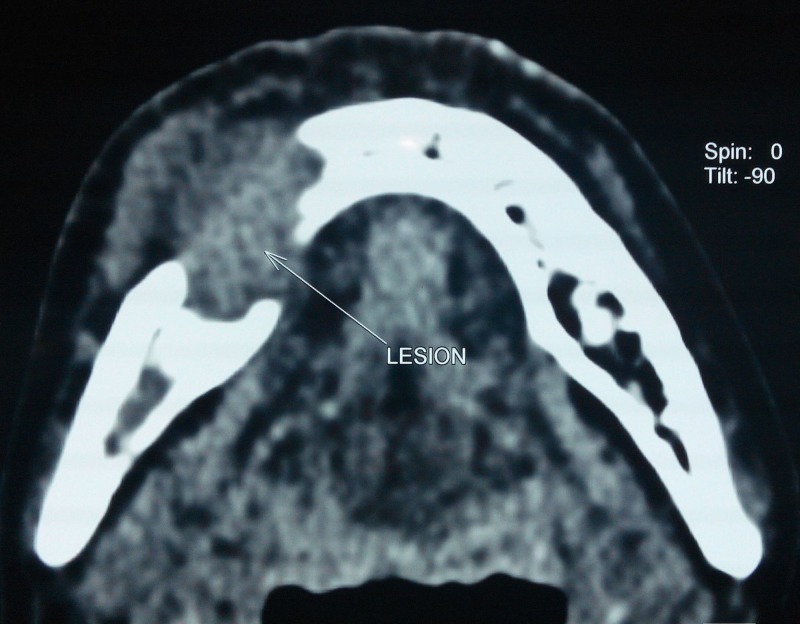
Axial section of plain computed tomographic image showing a 4.4 × 3.7 × 2.6 cm size ill-defined well-enhancing soft tissue density lesion at anterior aspect of body of mandible, gingival mucosa, right lower gingiva-buccal sulcus and buccal mucosa, causing lysis of mandible with erosion involving both buccal and lingual cortex with loss of fat plane between the lesion and right mylohyoid muscle.
